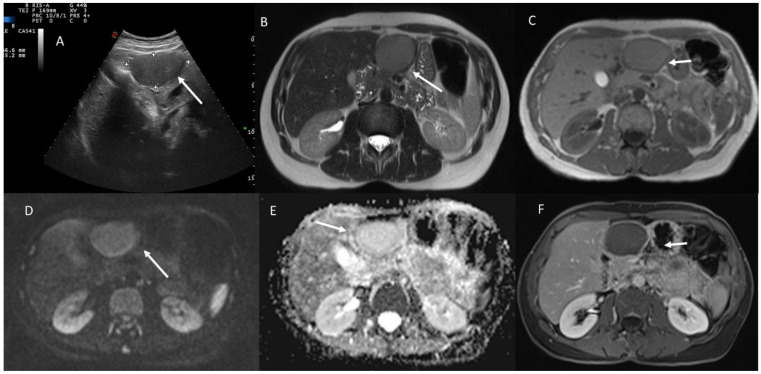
US (A) and MRI (B–F) assessment of cystadenoma patient. Upon US (A), the lesion shows solid appearance (white arrow), while in T2-weigthed sequences (B) and T1-weighted imaging (C): in phase) it shows non-solid pattern (white arrows) with restricted diffusion (D): b800 s/mm^2^ and (E): Apparent Diffusion Coefficient map) and peripheral contrast enhancement (white arrow) in T1-weigthed sequence in portal phase of contrast study.
